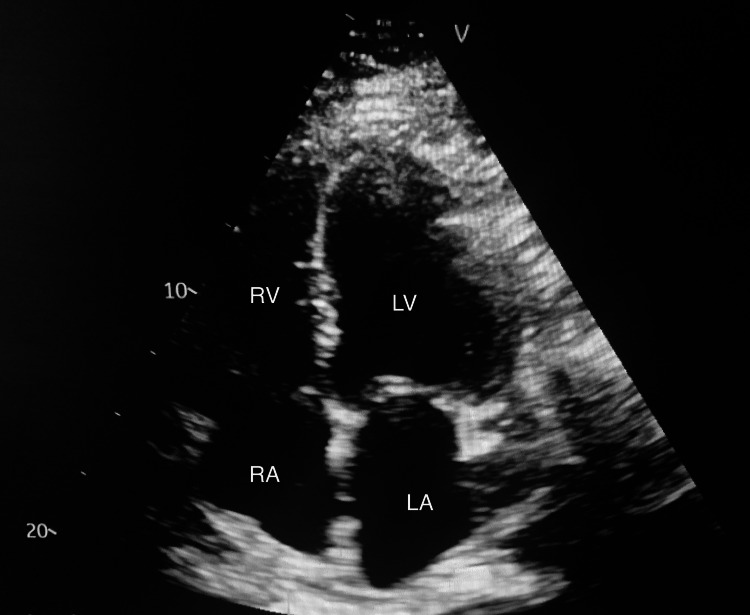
TTE (apical 4 chamber view) showing dilated cardiac chambers including mild dilation of the left ventricle (LV), right atrium (RA), and right ventricle (RV), and moderate dilation of the left atrium (LA). The ejection fraction was estimated at 10% with severely impaired left ventricular systolic function, impaired right ventricular systolic function, and grade three diastolic dysfunction. TTE: Transthoracic echocardiogram.
